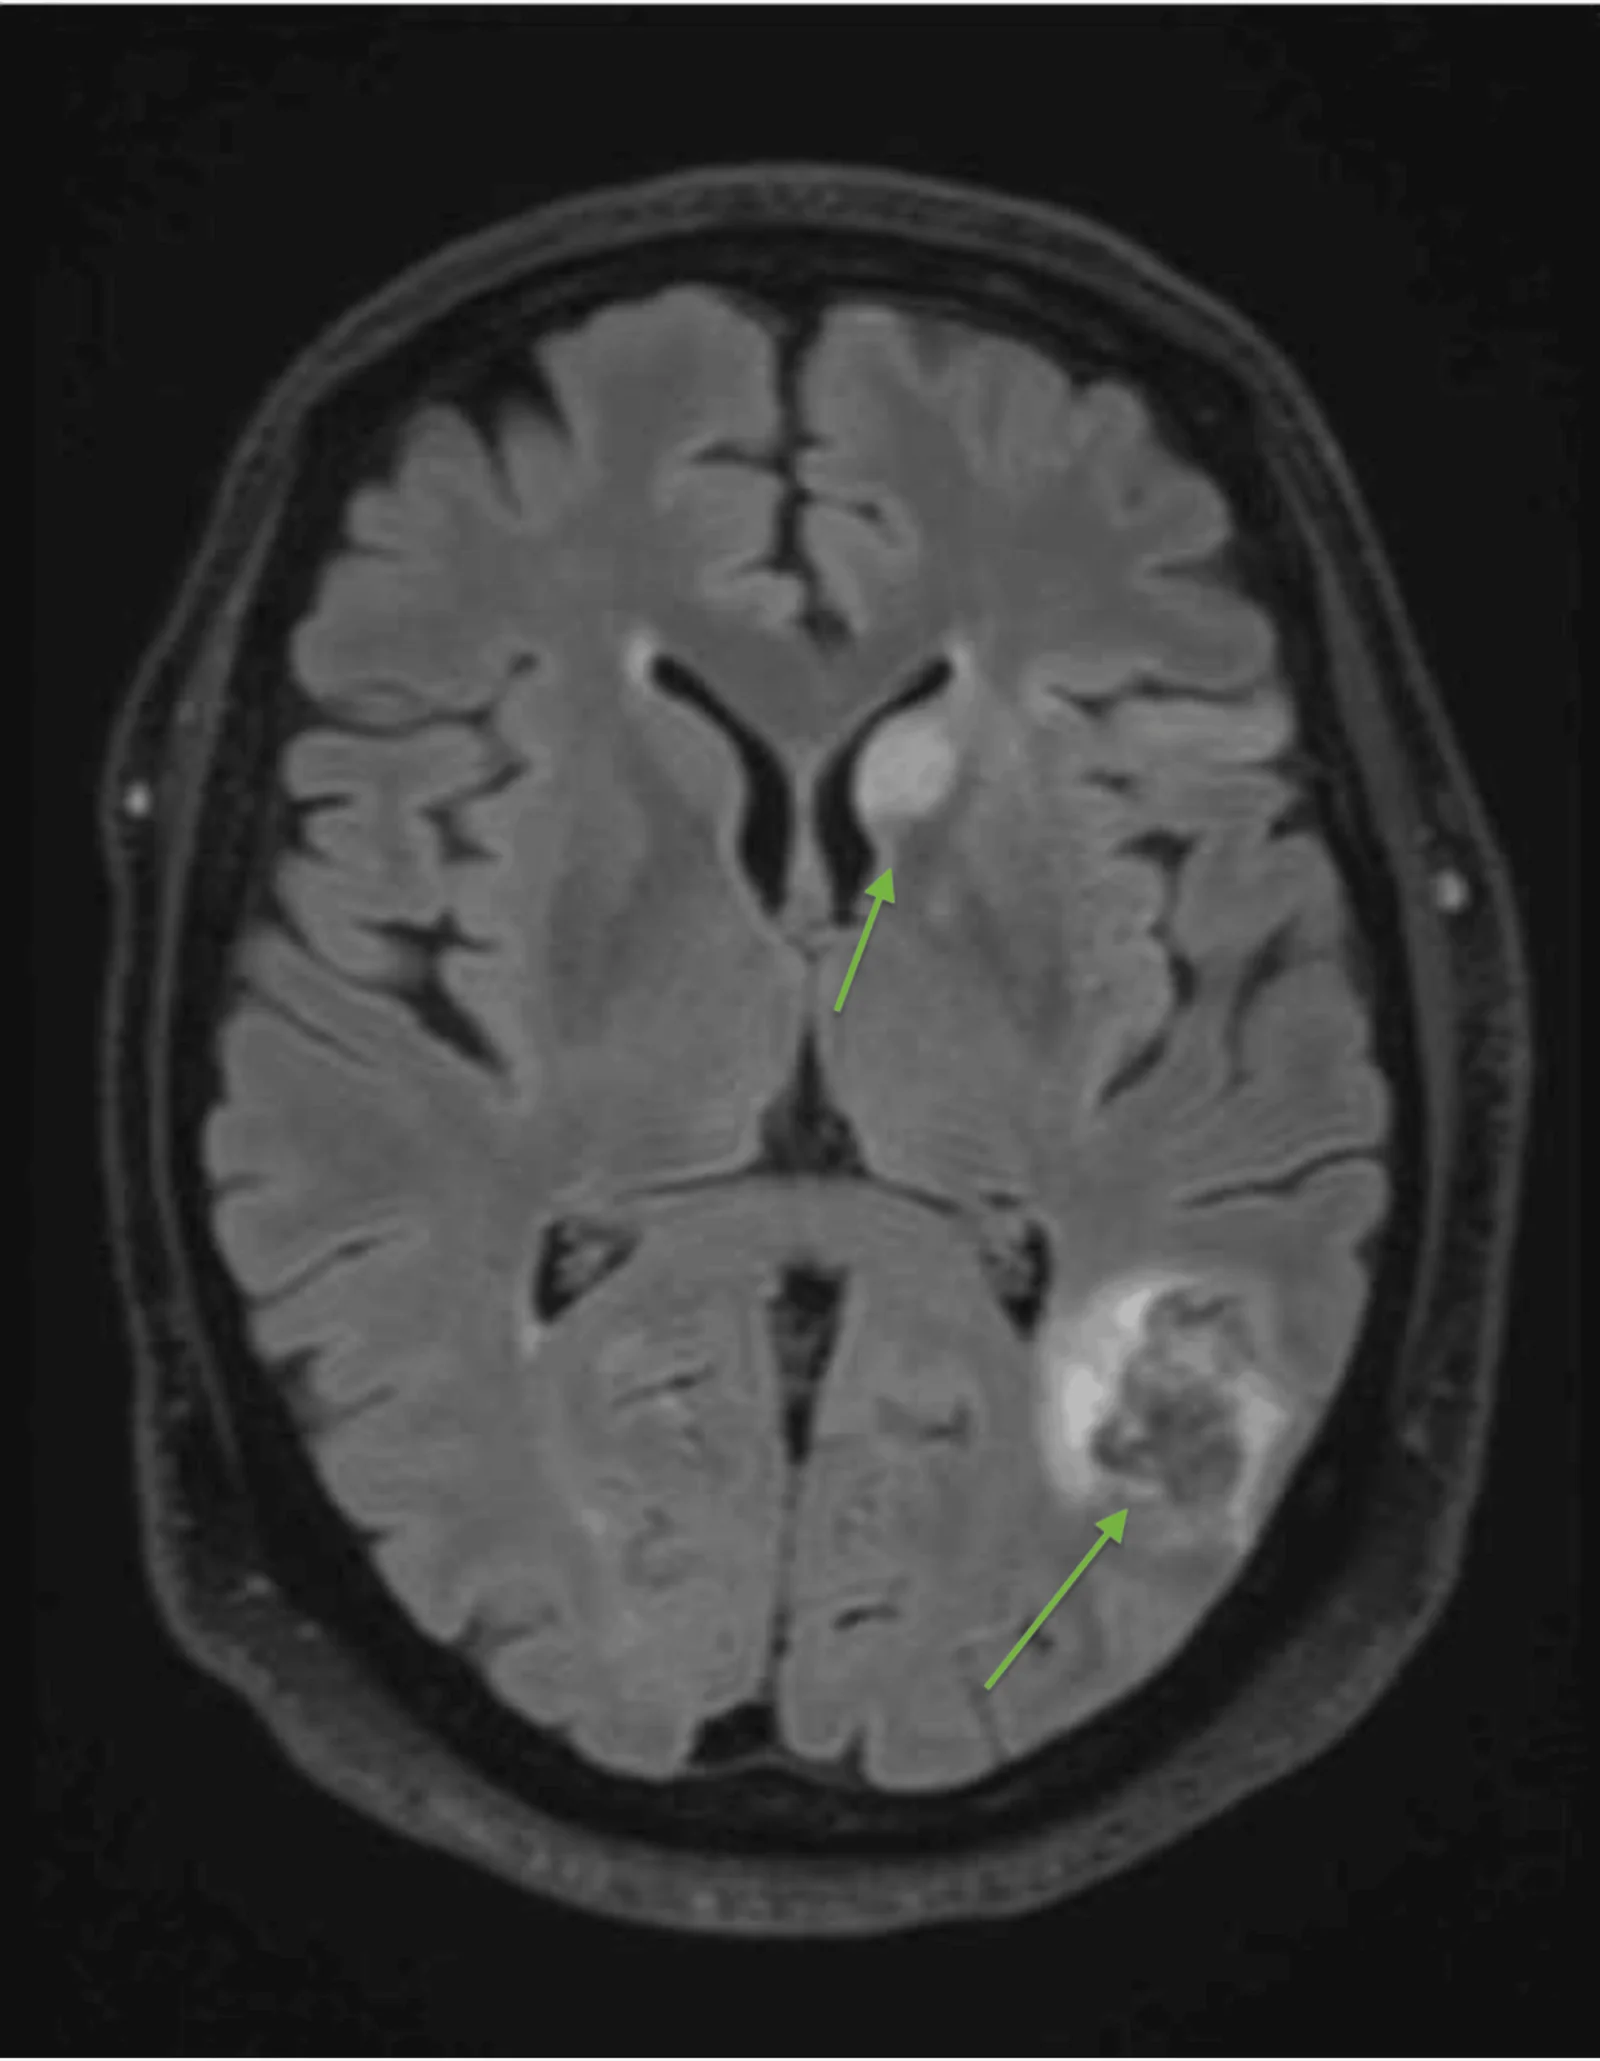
T2-weighted brain MRI showing multiple cortical ischemic lesions on the right and left cerebral hemispheres MRI: magnetic resonance imaging
